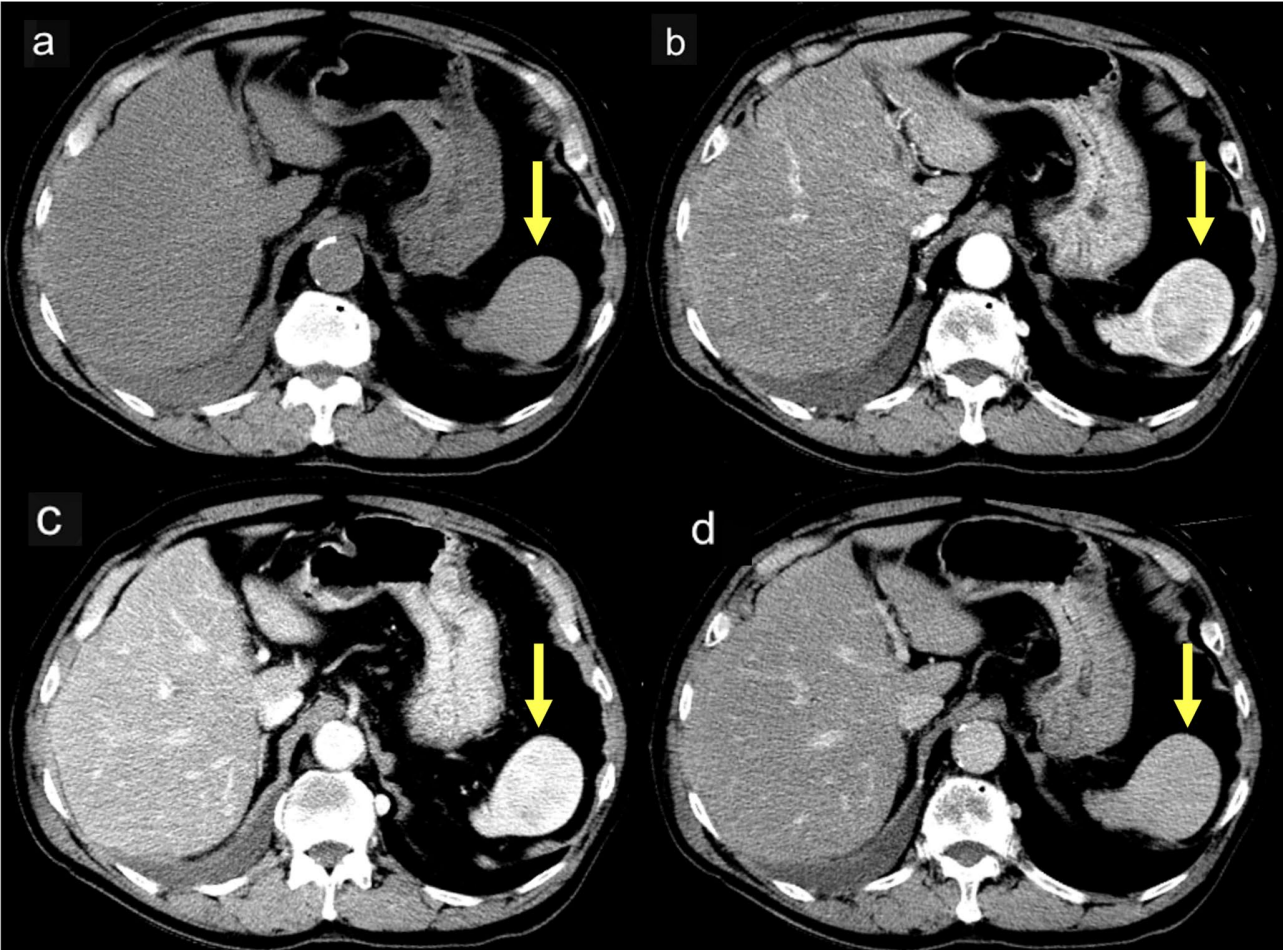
a Non-contrast CT reveals a well-defined splenic mass, isoattenuating to the surrounding splenic parenchyma. b Arterial phase of contrast-enhanced CT demonstrates peripheral-predominant, heterogeneous enhancement. c Portal venous phase of contrast-enhanced CT reveals progressive centripetal enhancement with mild residual heterogeneity. d Delayed phase of contrast-enhanced CT demonstrates near-homogeneous attenuation approximating that of the surrounding parenchyma. The lesion is indicated by yellow arrows. CT: computed tomography
